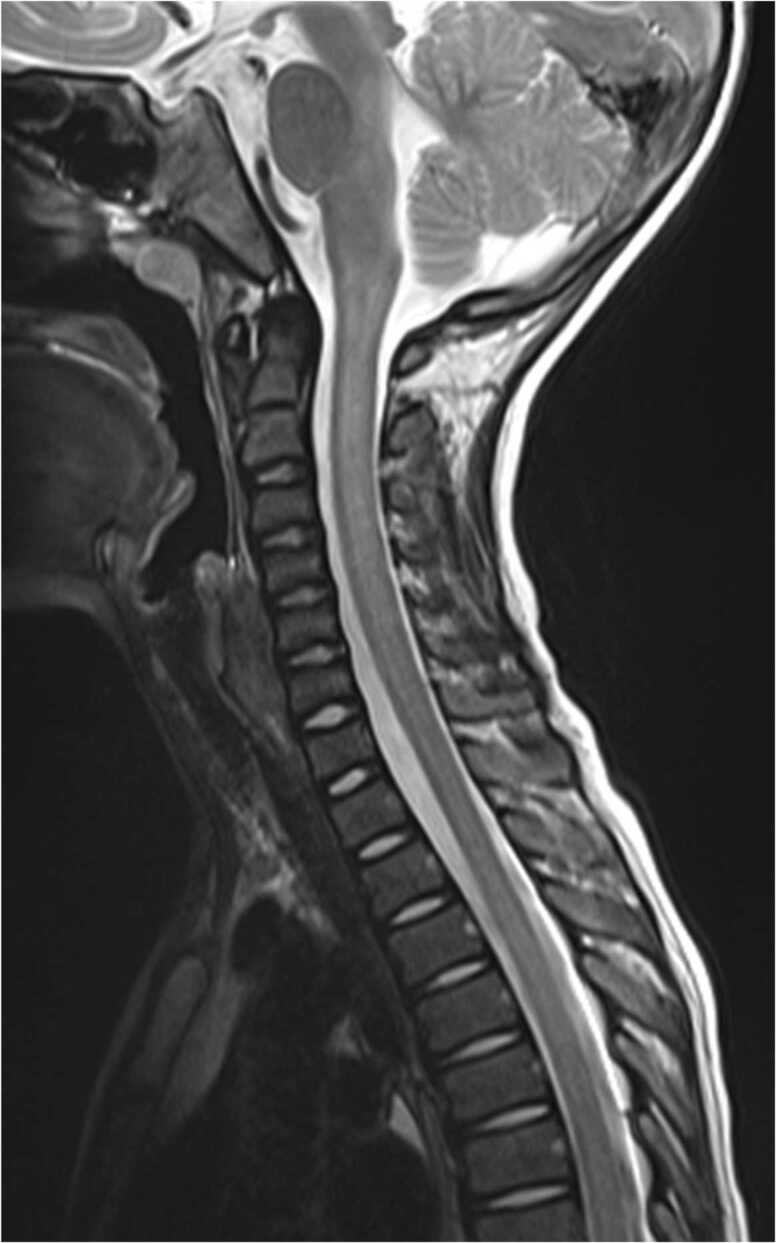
T2-weighted image (T2WI) sagittal image of the cervical and thoracic spine, showing T2WI hyperintense longitudinally extensive lesion in the central gray matter.
